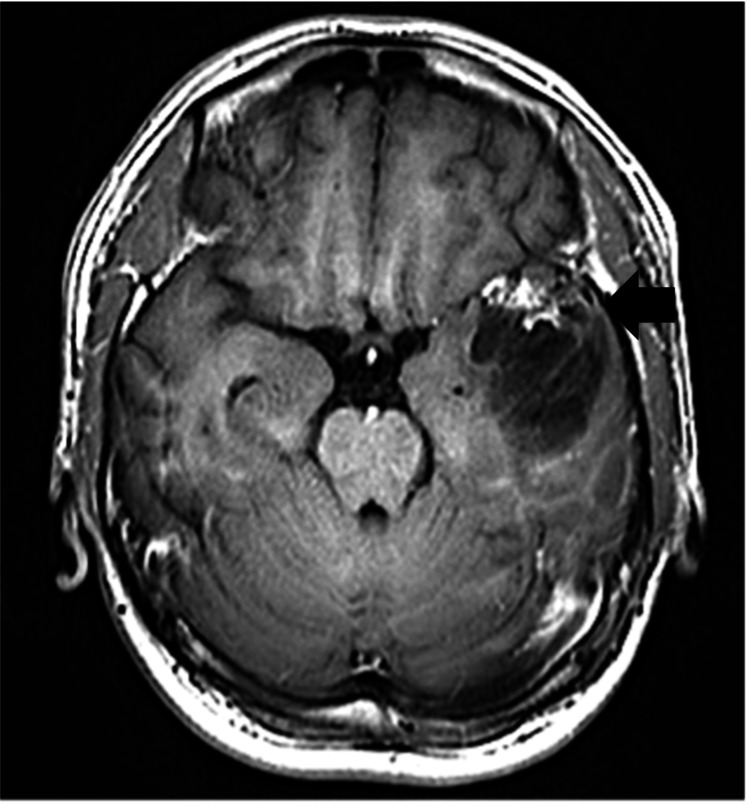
Axial magnetic resonance image showing a Gd-DTPA-enhanced lesion (black arrow) with perifocal edema appeared in the tip of the left temporal lobe. Gd-DTPA, gadolinium-diethylenetriaminepentaacetic acid
